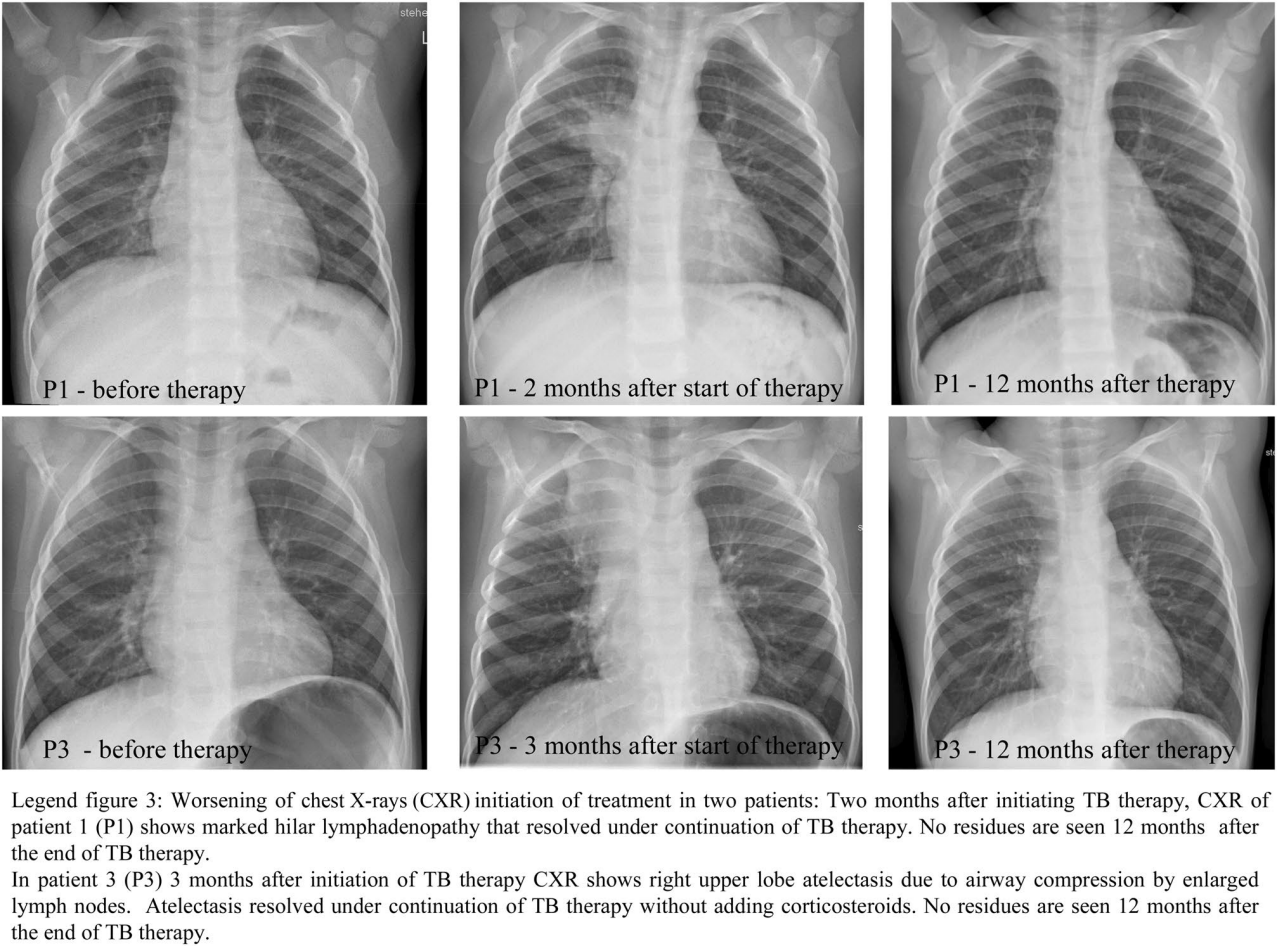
Worsening of chest X-rays after initiation of treatment (paradoxical reaction)
